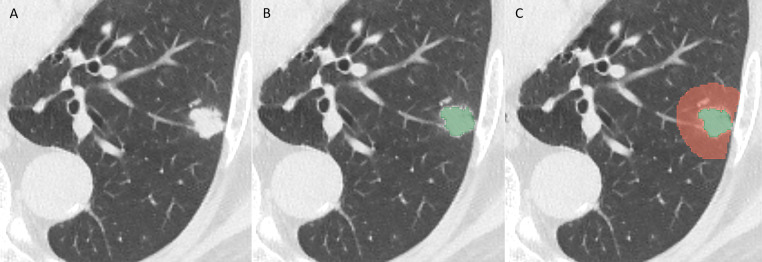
Volume of interest (VOI) segmentation of the lesions. (A) An irregular nodule on the non-enhanced CT image. (B) The lesion volume (*green region*) was manually delineated slice by slice on lung windows. (C) It was automatically expanded outward by 10 mm from the nodular edge, and the lesion components and structures such as bones were manually removed to obtain the perilesional volume (*red region*).
